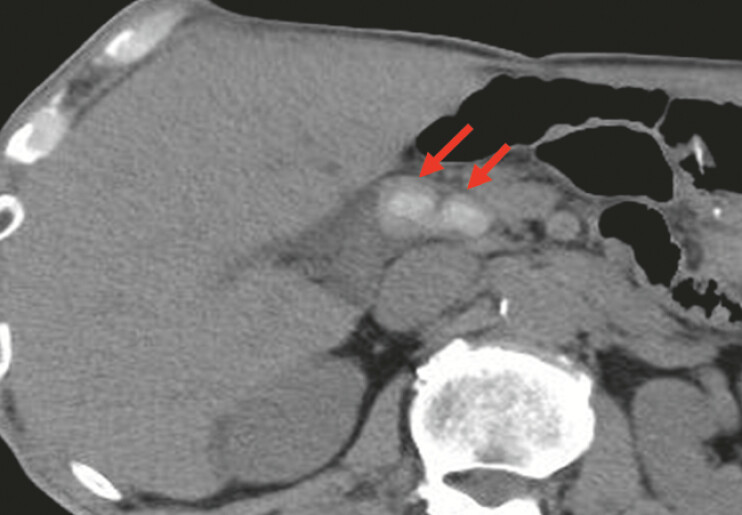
Computed tomography revealing multiple large stones in the common bile duct (red arrow).
